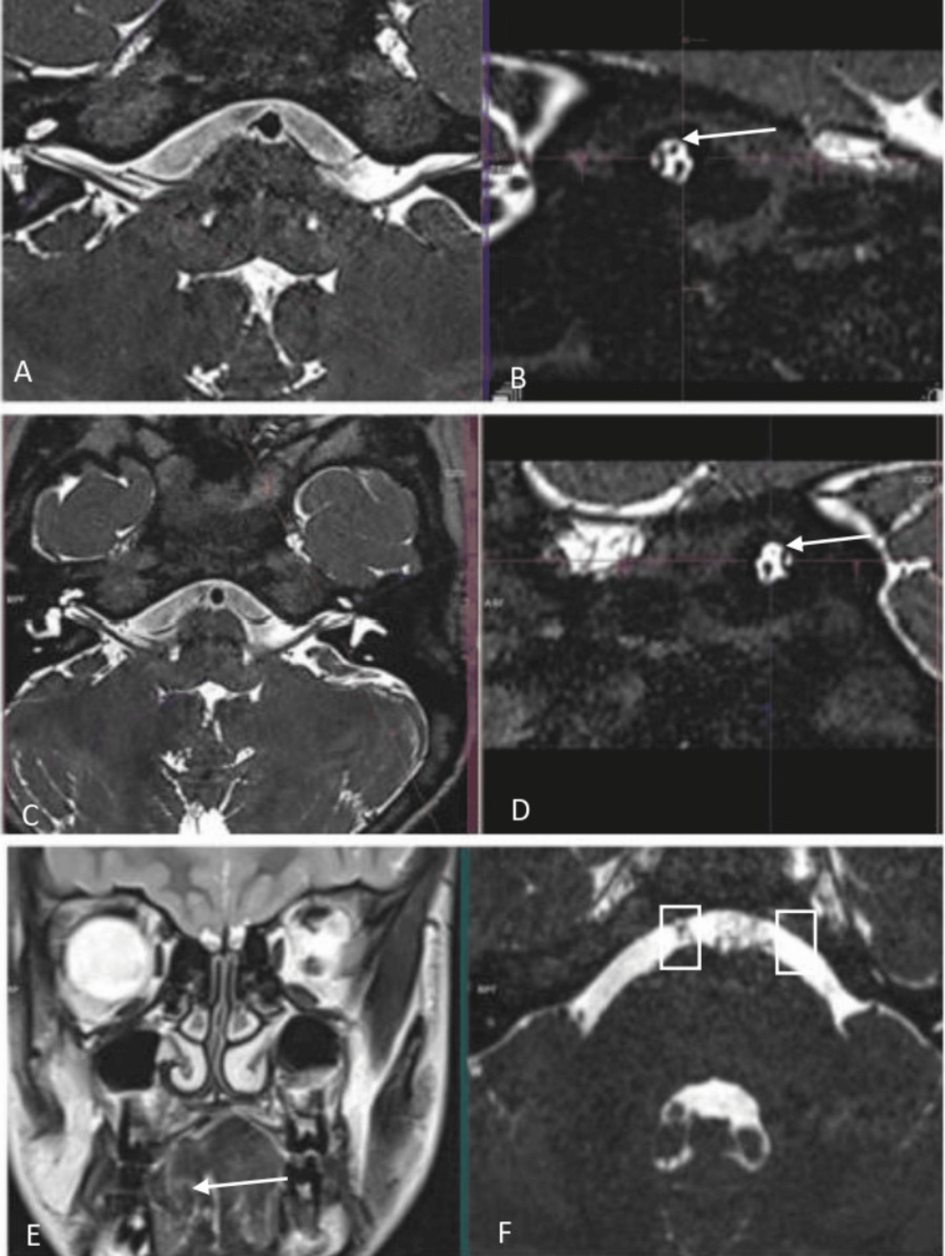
Axial (A) and oblique sagittal (B) 3D SPACE T2-weighted MRI images show the normal right facial nerve, located in the anterior superior quadrant of the internal auditory canal. (C, D) Marked hypoplasia of the left facial nerve is seen (arrow), while the right facial nerve appears normal. (E) Volume loss in the right half of the tongue (arrow), likely secondary to right hypoglossal nerve palsy. (F) A linear structure consistent with the right abducens nerve is visible (rectangle), while the left abducens nerve is not appreciable, suggesting congenital absence. CSF flow artifact is noted in the prepontine cistern. SPACE: Sampling Perfection with Application-optimized Contrasts using different flip-angle Evolutions
